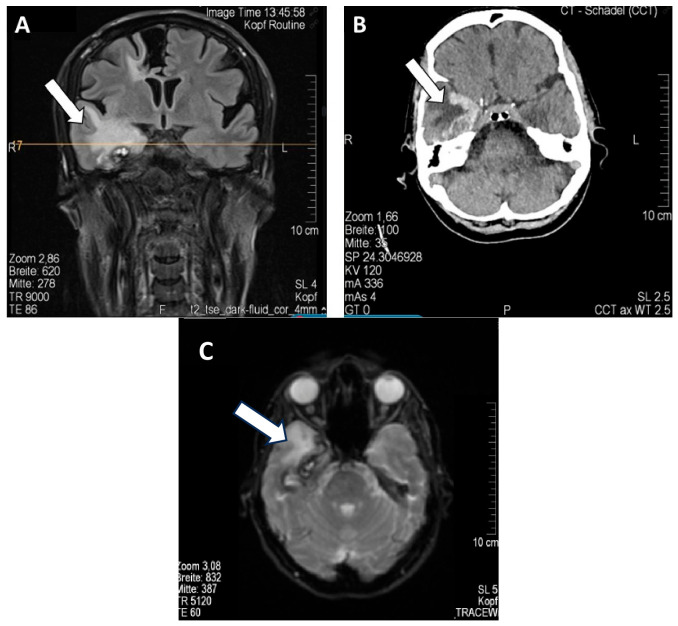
FLAIR-weighted MRI of patient 2 showing a right temporal hyperintensity as a correlate of HSVE ((A) arrow). Cranial CT shows a right temporal hyperdensity (hemorrhage) within a hypodense region correlating to cerebral edema ((B) arrow). DWI-weighted MRI demonstrates diffusion restriction within the lesion ((C) arrow).
